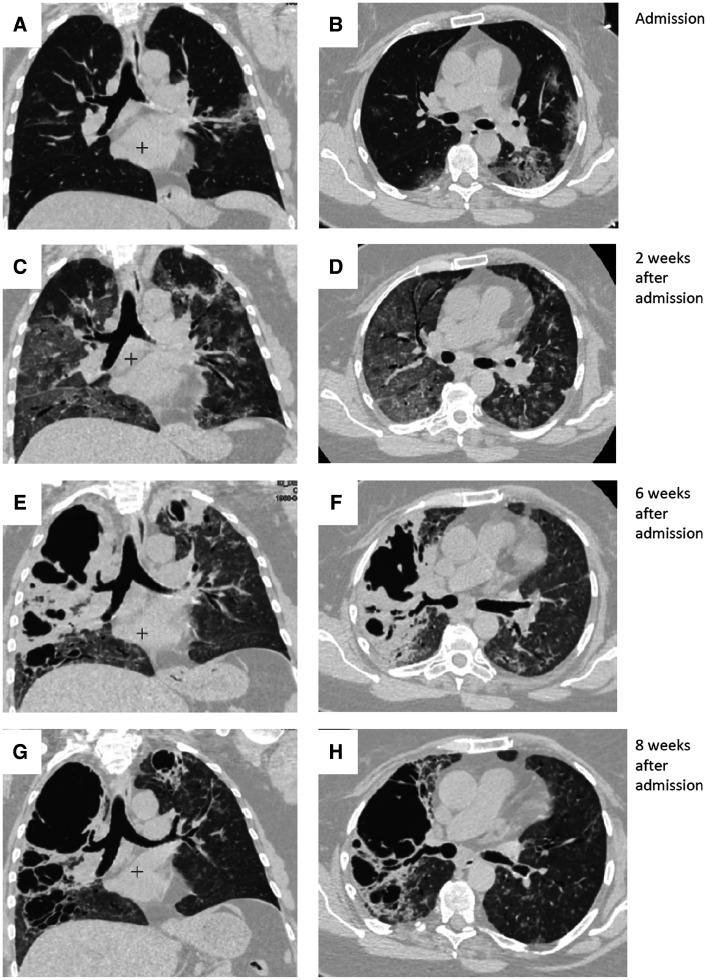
Thoracic computed tomographic scans (A, B) at admission showing diffuse bilateral ground-glass opacities predominantly involving the left lower lobe; (C, D) 2 weeks after admission showing diffuse subtotal bilateral ground-glass attenuation and linear opacities; (E, F) 6 weeks after admission showing extensive bilateral thick-walled, irregularly shaped cavities, with several coalescing into a larger one; and (G, H) 8 weeks after admission showing persistent bilateral cavitary lesions. (A, C, E, G) Coronal views. (B, D, F, H) Axial views below the carina.
